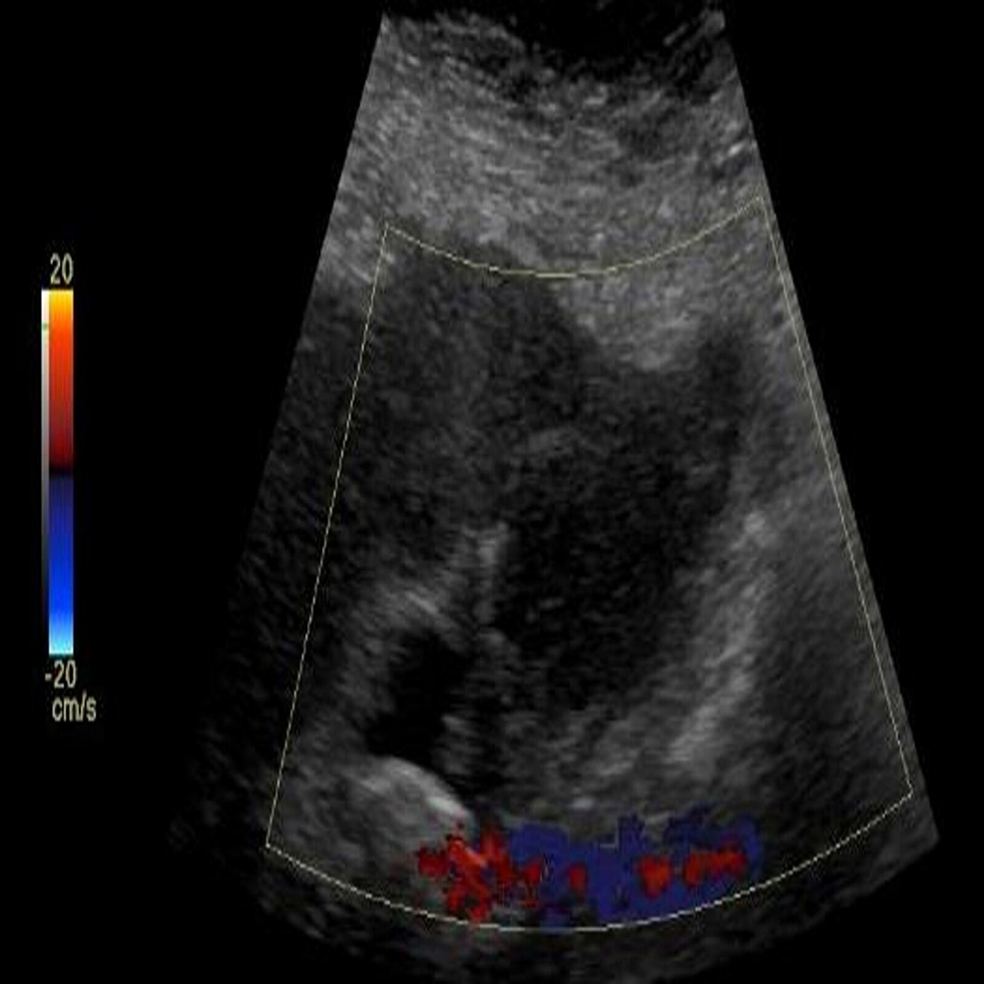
Ultrasound of the gallbladder showing enlarged gallbladder, with thickened irregular wall.
